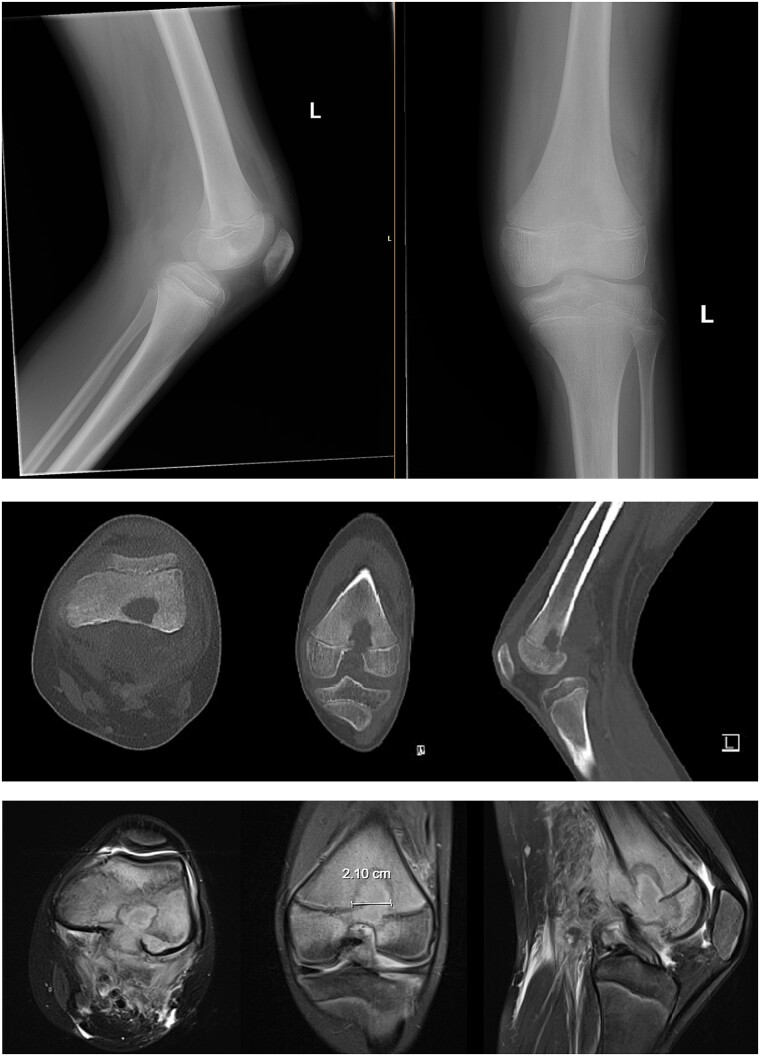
Preoperative radiography, CT, and MRI. The radiographs were read as normal by the radiology, while the CT and MRI were read osteomyelitis with Brodie’s abscess involving the distal femoral epimetaphysis with destruction of the posterior cortex and associated 7 cm popliteal fossa abscess, along with a suspected nonocclusive septic thrombophlebitis of the popliteal vein mentioned in the report on the CT.
